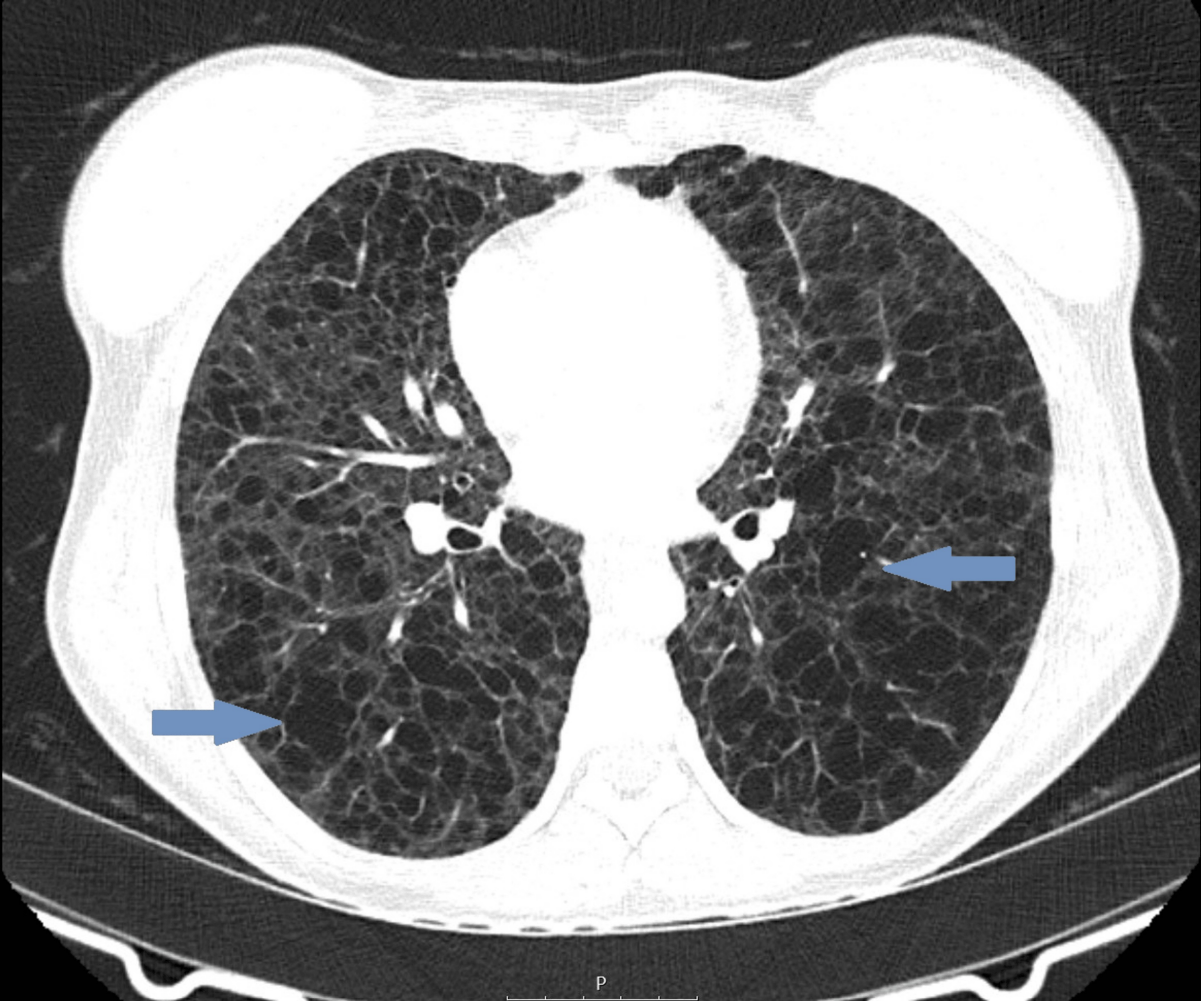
Axial view of thoracic computed tomography scan showing multiple thin-walled intrapulmonary cysts with a diffuse distribution, suggestive of lymphangioleiomyomatosis (arrows).
